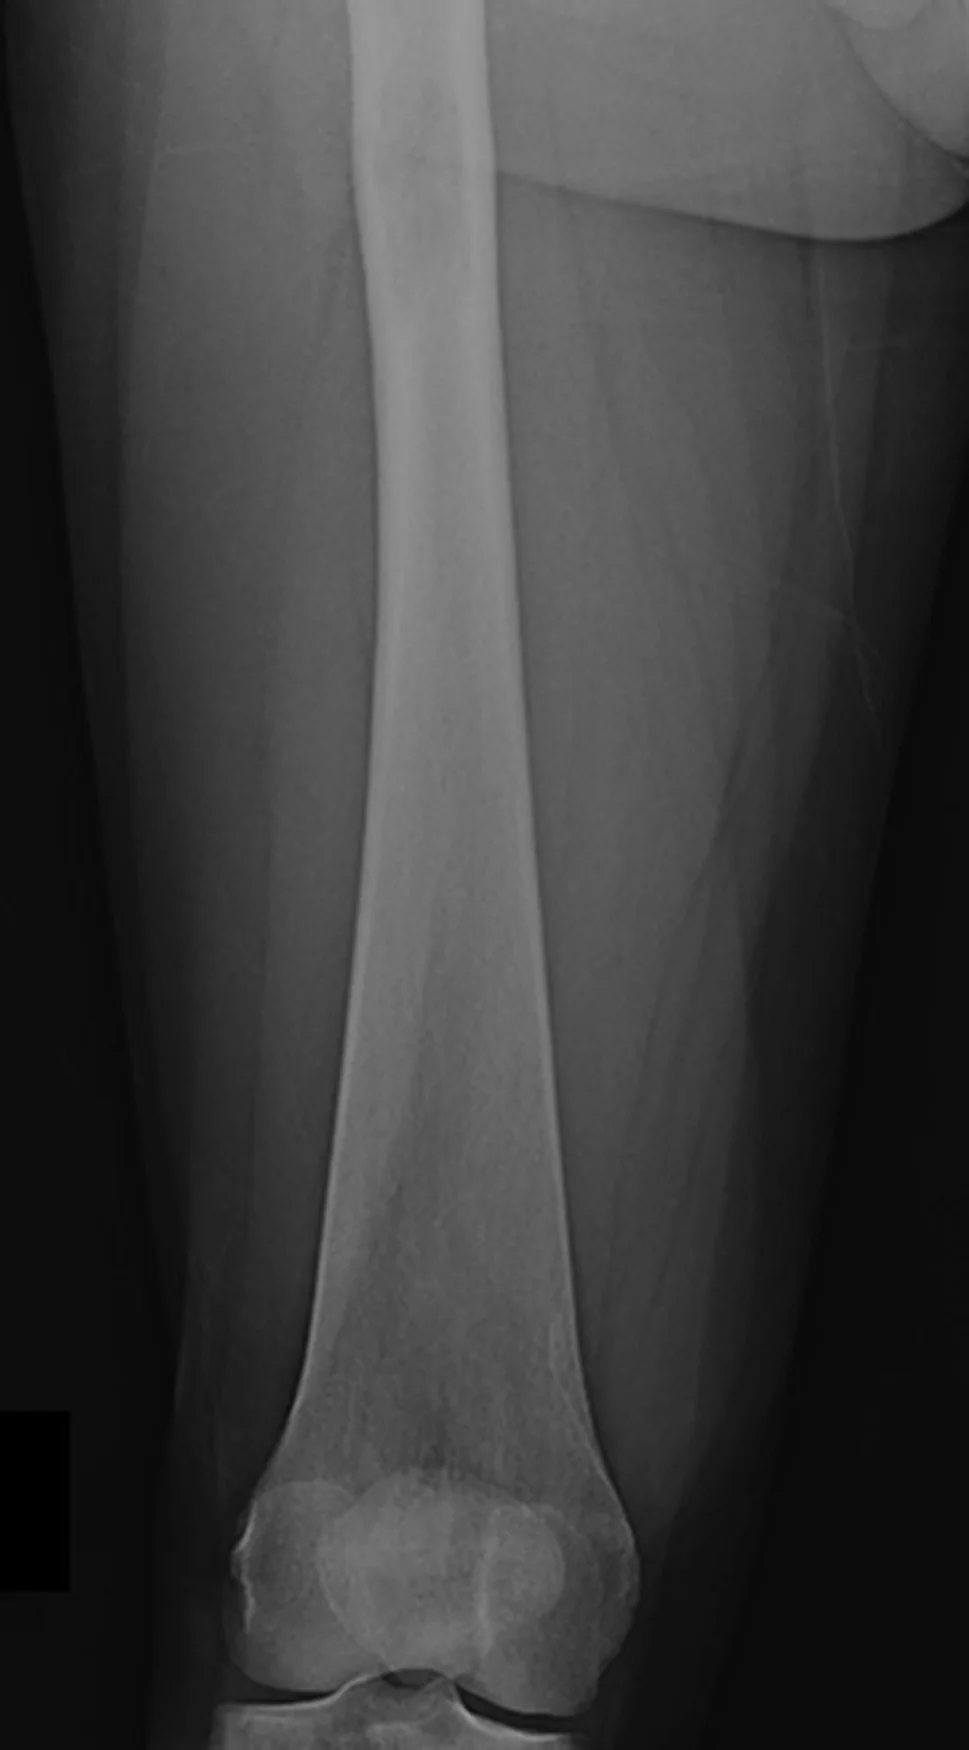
Plain radiograph of the right distal femur of a patient with Gaucher disease under enzyme replacement therapy. Note the Erlenmeyer flask deformity with enlargement of the metaphyseal region. Courtesy of Dr. Diogo Souza Domiciano
